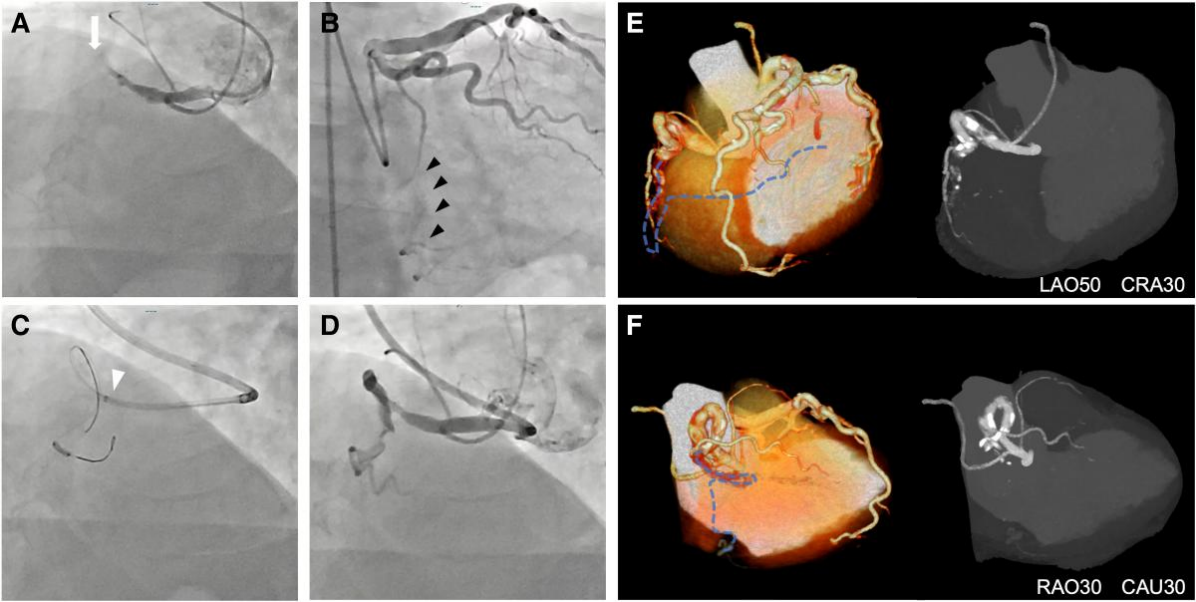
Coronary angiography and cardiac computed tomography angiography of this case. (*A*) Emergency angiography demonstrated thromboembolic occlusion of the right coronary artery (*white arrow*). (*B*) The atrial branch of the circumflex artery supplied the distal right coronary artery via a severely tortuous collateral artery (*black arrowheads*). This collateral artery could not be used for a retrograde approach. (*C*) The side branch balloon anchoring technique was unsuccessful for guidewire penetration of the chronic total occlusion due to insufficient backup force (*white arrowhead*). (*D*) The proximal right coronary artery was recanalized by thrombectomy. (*E* and *F*) Computed tomography angiography demonstrated the long chronic total occlusion lesion with sparse mural calcification. The dotted blue line represents the estimated vessel course, showing a severely tortuous occluded lesion in the distal right coronary artery segment.
